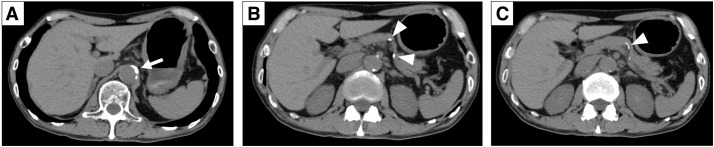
Preoperative contrast-enhanced CT images. (A–C) Severe arterial calcification of the abdominal aorta (arrow) and splenic artery (arrowheads).
